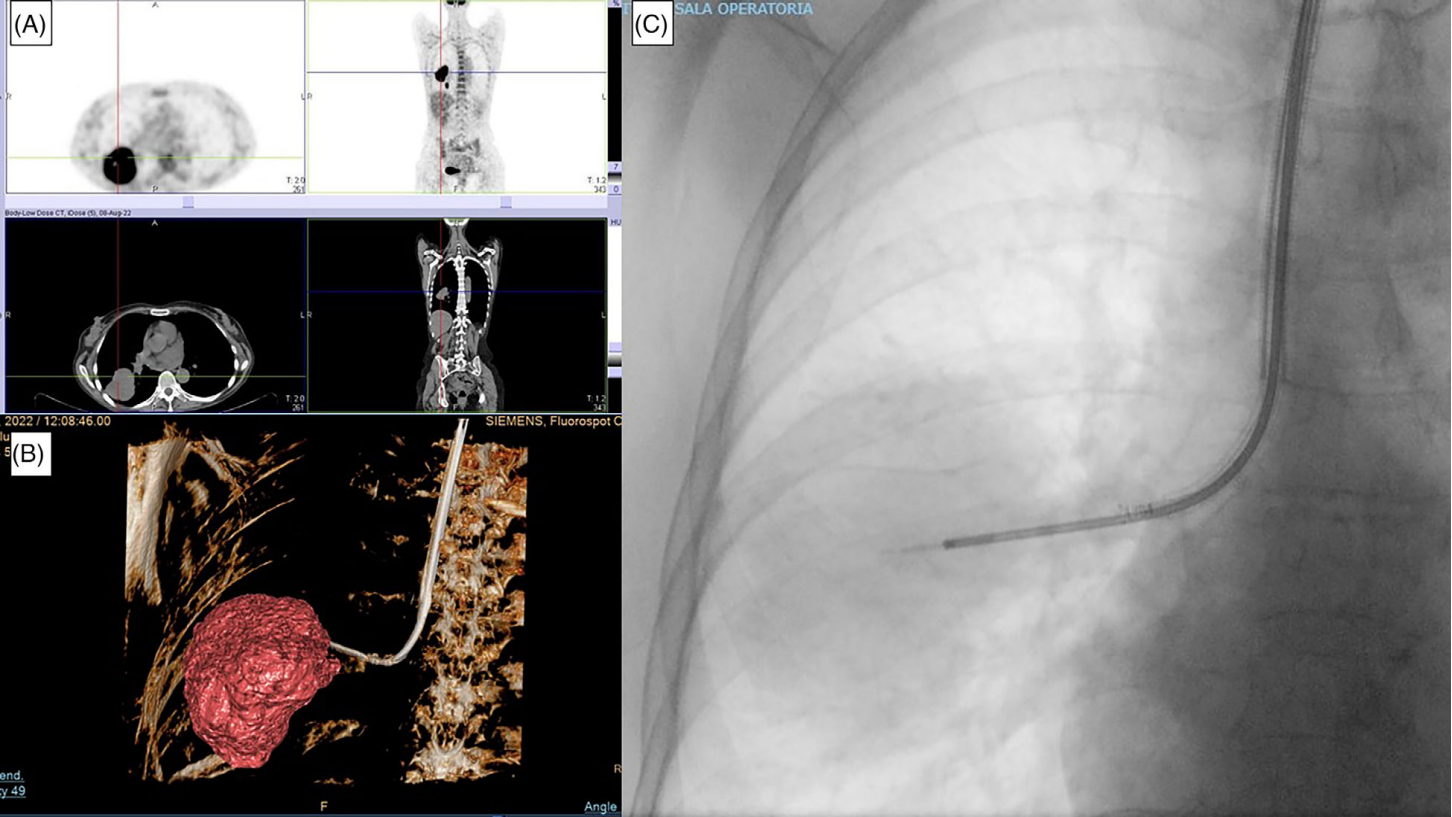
(A) 18F‐fluorodeoxyglucose (FDG) PET/CT uptake in correspondence to the right B6 segment; (B) aScope 5 Broncho HD 5.6/2.8 single‐use bronchoscope reaches the lesion guided by 3D fluoroscopy CIOS guidance system; C. fluoroscopic image of TBNA sampling of the lesion.
